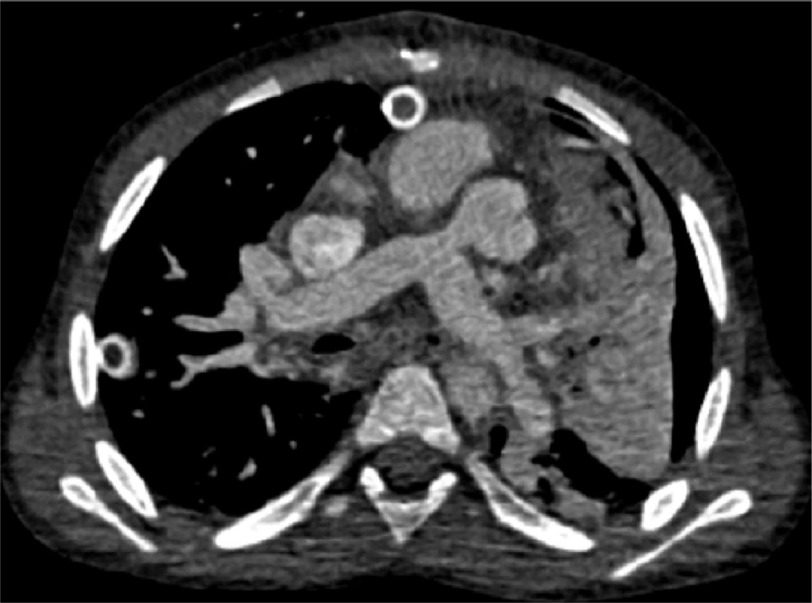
Early post-operative CT image showing the degree of tightness of the pulmonary artery band.
